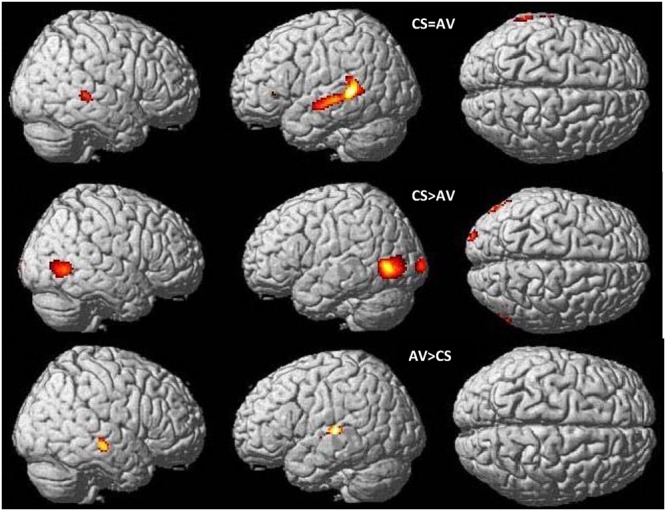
Common and distinct patterns in CSLM and speech AV during word perception. Conjunction analysis between speech AV [speech AV – still] and CSLM [CSLM – still] perception of words (top). Higher activations during CSLM perception of words [CSLM – still] compared to speech AV perception of words [speech AV – still] (middle) and during speech AV perception of words [speech AV – still] compared to CSLM perception of words [CSLM – still] (bottom). Conjunction analysis and CSLM > speech AV activations are displayed at *p* < 0.05 whole brain corrected while speech AV > CSLM is displayed at *p* < 0.001 uncorrected. All are superimposed on the SPM MRI template. CSLM, Cued Speech (oral + manual) in deaf early users; CSM, Cued Speech (manual only); AV, audiovisual speech in hearing speakers.
